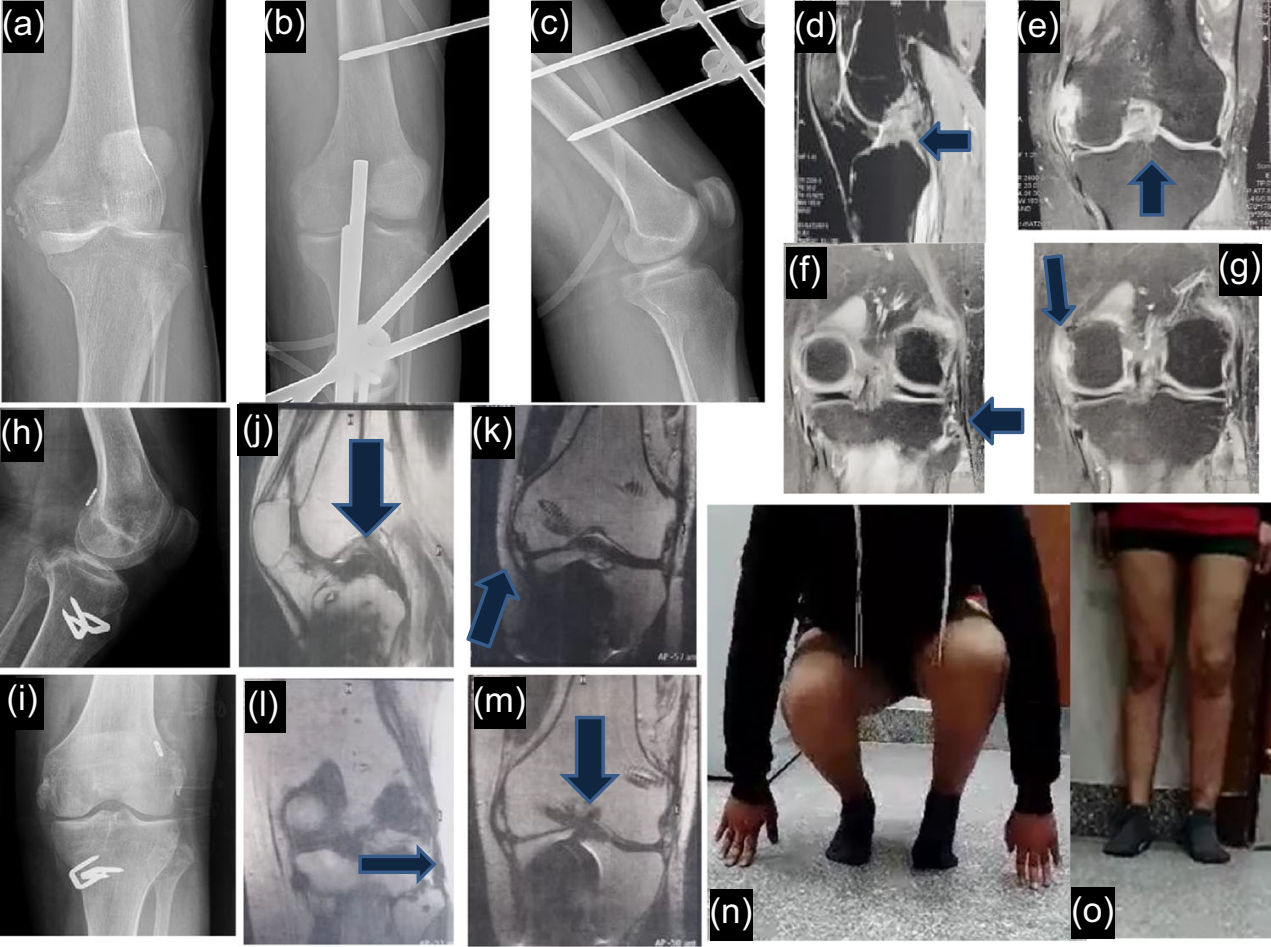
(a) Preoperative x‐ray AP view of the dislocated knee. (b & c) Postoperative x‐ray AP and Lateral views of knee after reduction with cross knee external fixator. (d–g) Preoperative fat‐suppressed coronal and sagittal MRI images showing PCL, ACL, PLC and MCL injury. (h & i) Two years postoperative follow up x‐ray AP and lateral views of the knee showing PCL, ACL and PLC reconstruction. (j–m): Two years postoperative follow‐up MRI knee showing PCL, ACL, MCL and PLC reconstruction. (n & o): Two years postoperative clinical photo of the knee range of motion. ACL, anterior cruciate ligament; MCL, medial collateral ligament; PCL, posterior cruciate ligament; PLC, posterolateral corner
